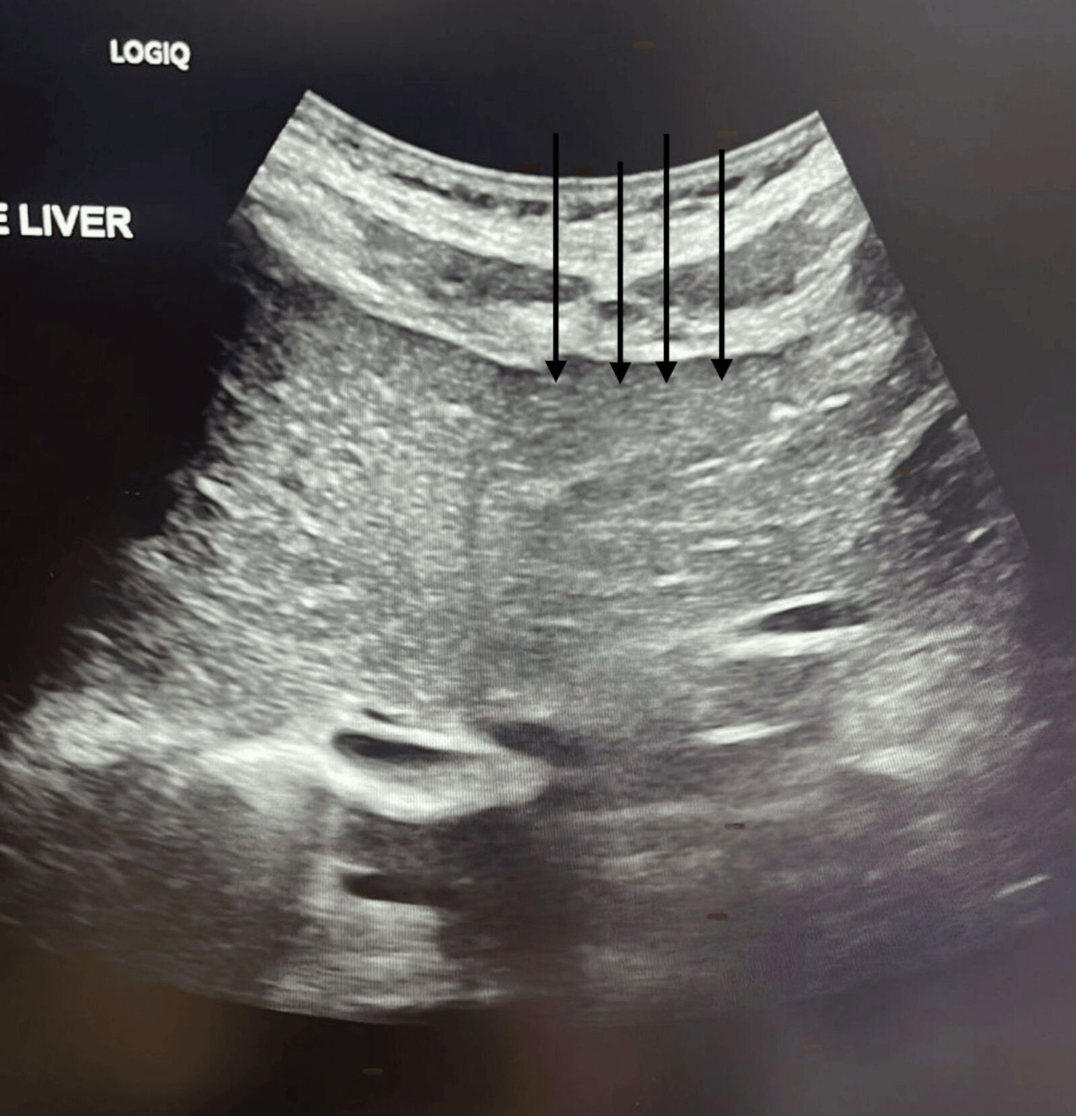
Ultrasound of the liver demonstrating cirrhotic changes. Nodular liver surface can be noted with no detectable hepatic focal lesion.
